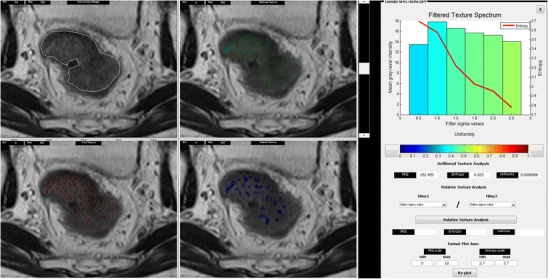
Texture analysis of a T2-weighted MRI image of rectal cancer
